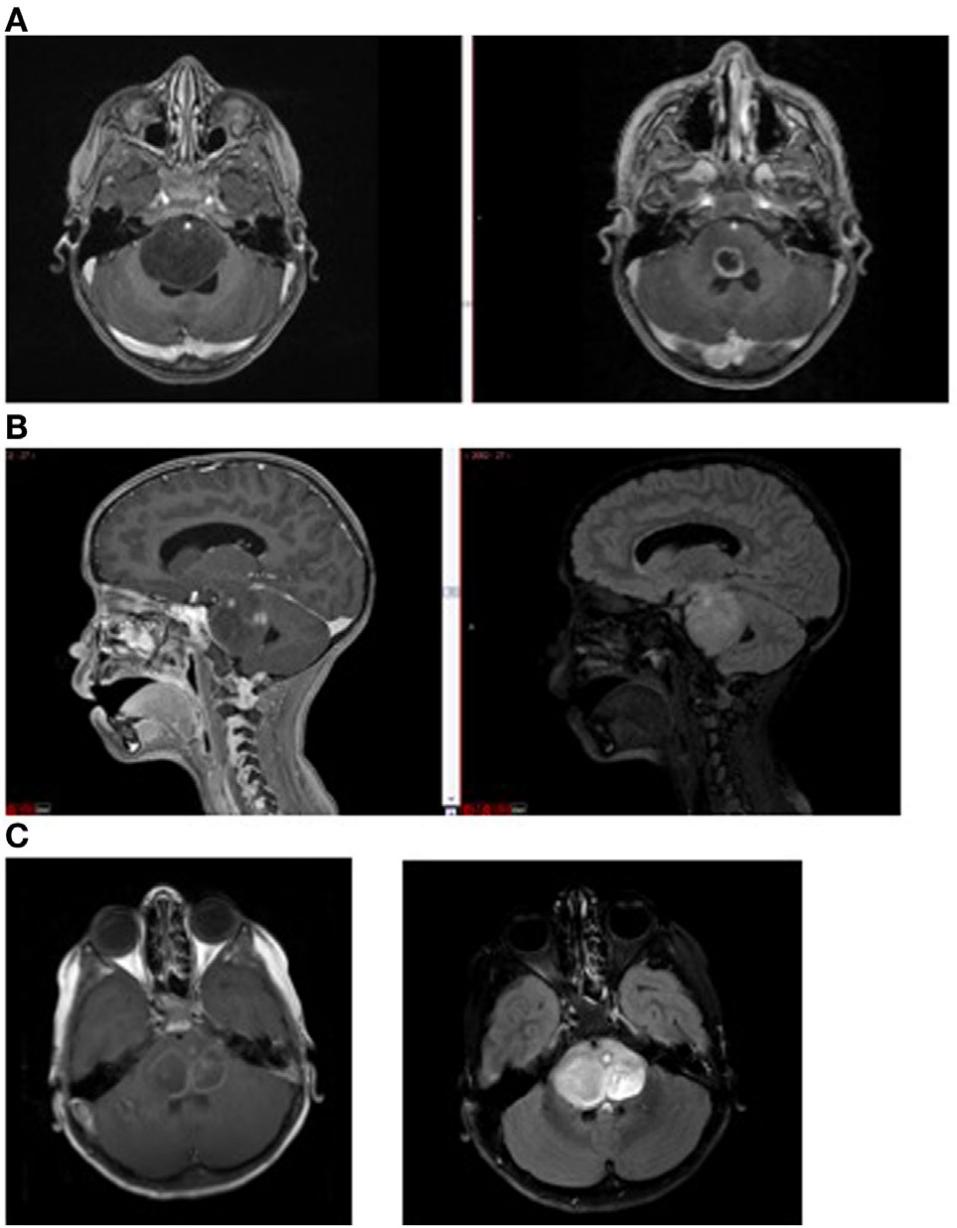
Magnetic resonance imaging demonstrating (A) non-enhancing tumor at diagnosis (left) and enhancement (right) following radiation therapy; (B) tumor necrosis (enhancement at diagnosis, left) and FLAIR image (right) showing extent of tumor beyond area of enhancement, and (C) intratumoral cysts. Areas of contrast enhancement do not represent tumor burden in diffuse intrinsic pontine glioma and do not ensure adequate drug delivery.
